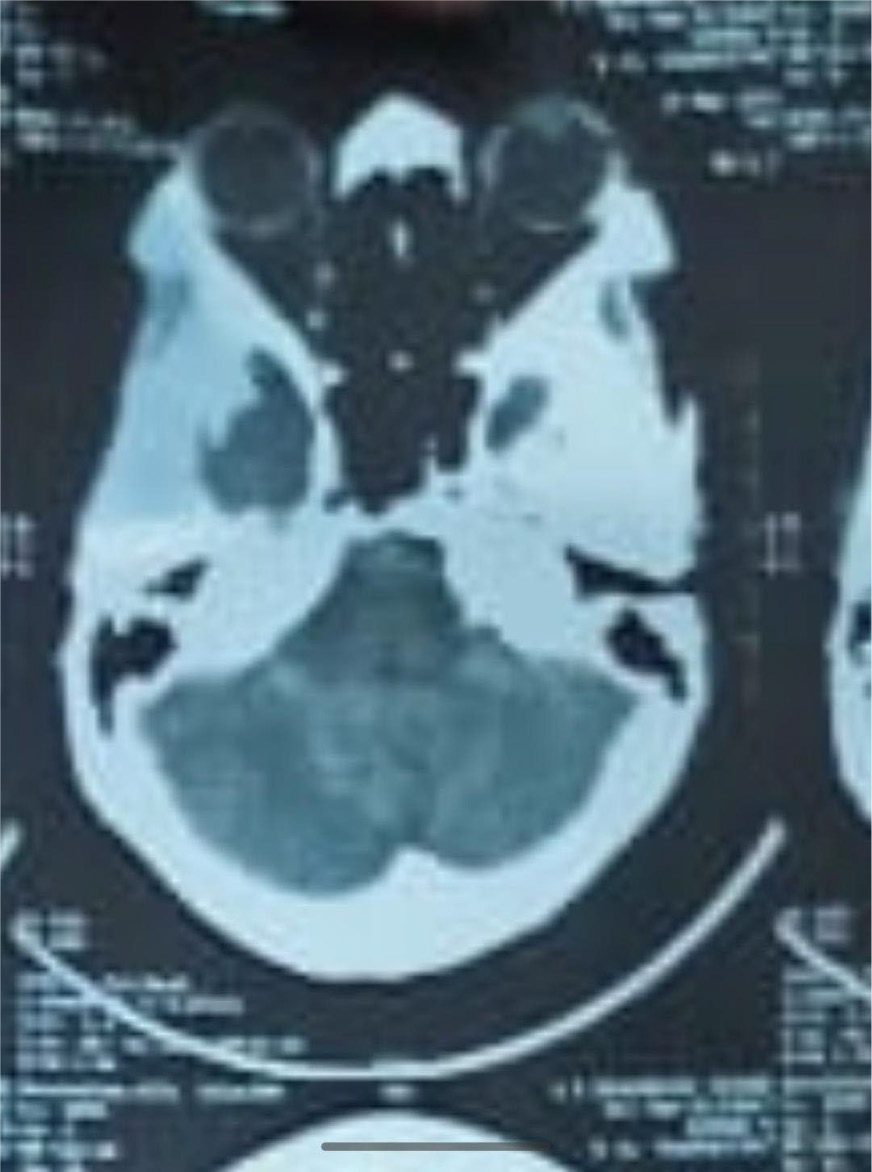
Axial section of CT Brain showing base of skull.
